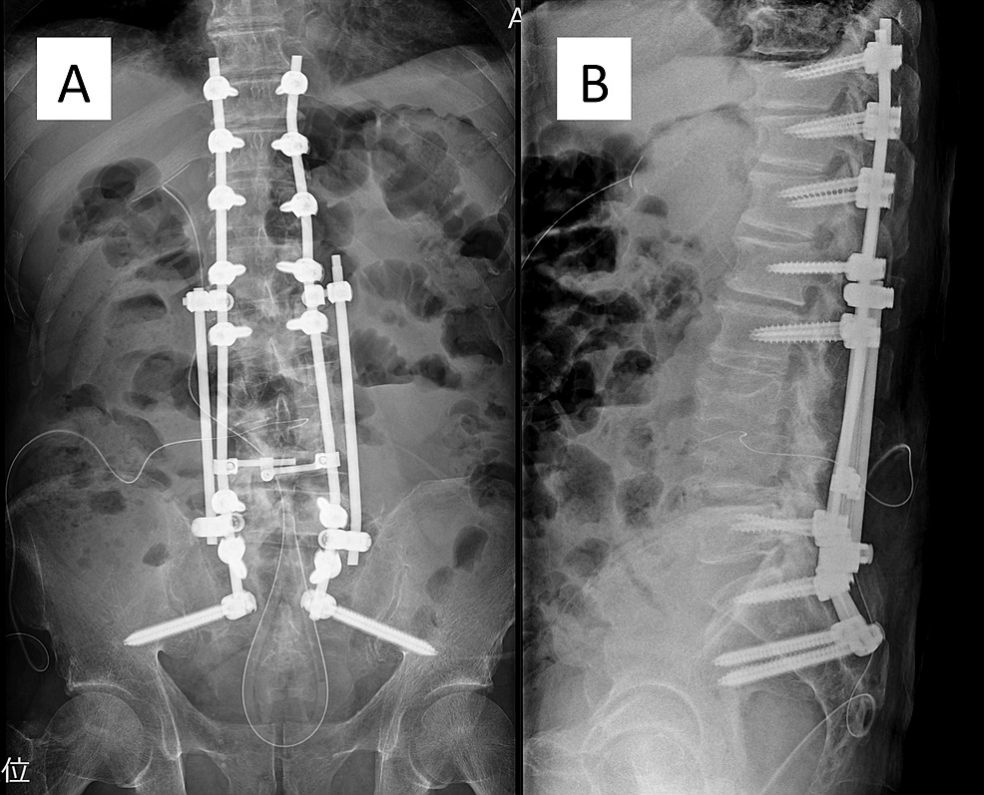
Postoperative X-ray images of posterior percutaneous screw fixation from T10 to the pelvis and percutaneous endoscopic discectomy/debridement to L3/4. (A) Anteroposterior X-ray image. (B) Lateral X-ray image.
